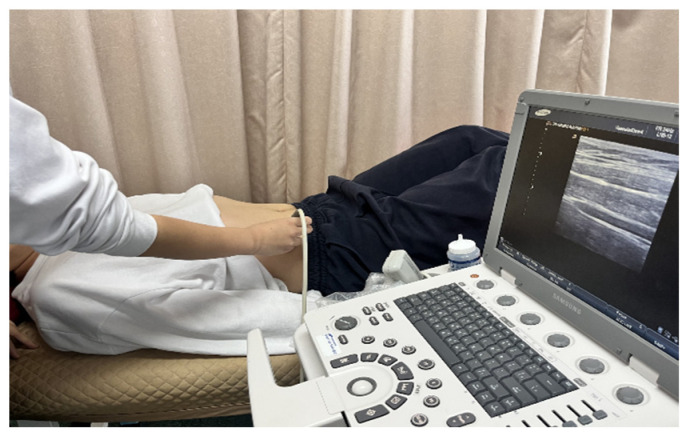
Ultrasound transducer placement over the lateral abdominal wall.
